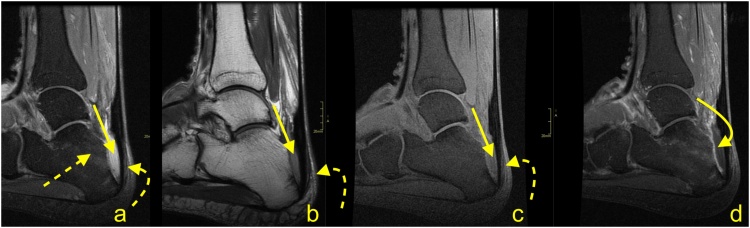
Fluid collection after surgery for Haglund's syndrome. Postoperative swollen heel (in an otherwise asymptomatic patient). MRI revealed a fluid collection (straight arrow) with discrete linear contrast wall enhancement (curved arrow), which indicates a seroma, and no evidence of abscess. Postoperative residual bone marrow edema (dashed arrow) in the calcaneus. Expected surgical thinning of the Achilles tendon is visible (curved dashed arrow). A- T2- weighted with fat suppression, b- PD- weighted, c-T1- weighted with fat suppression, d- T1- weighted with fat suppression and contrast.
